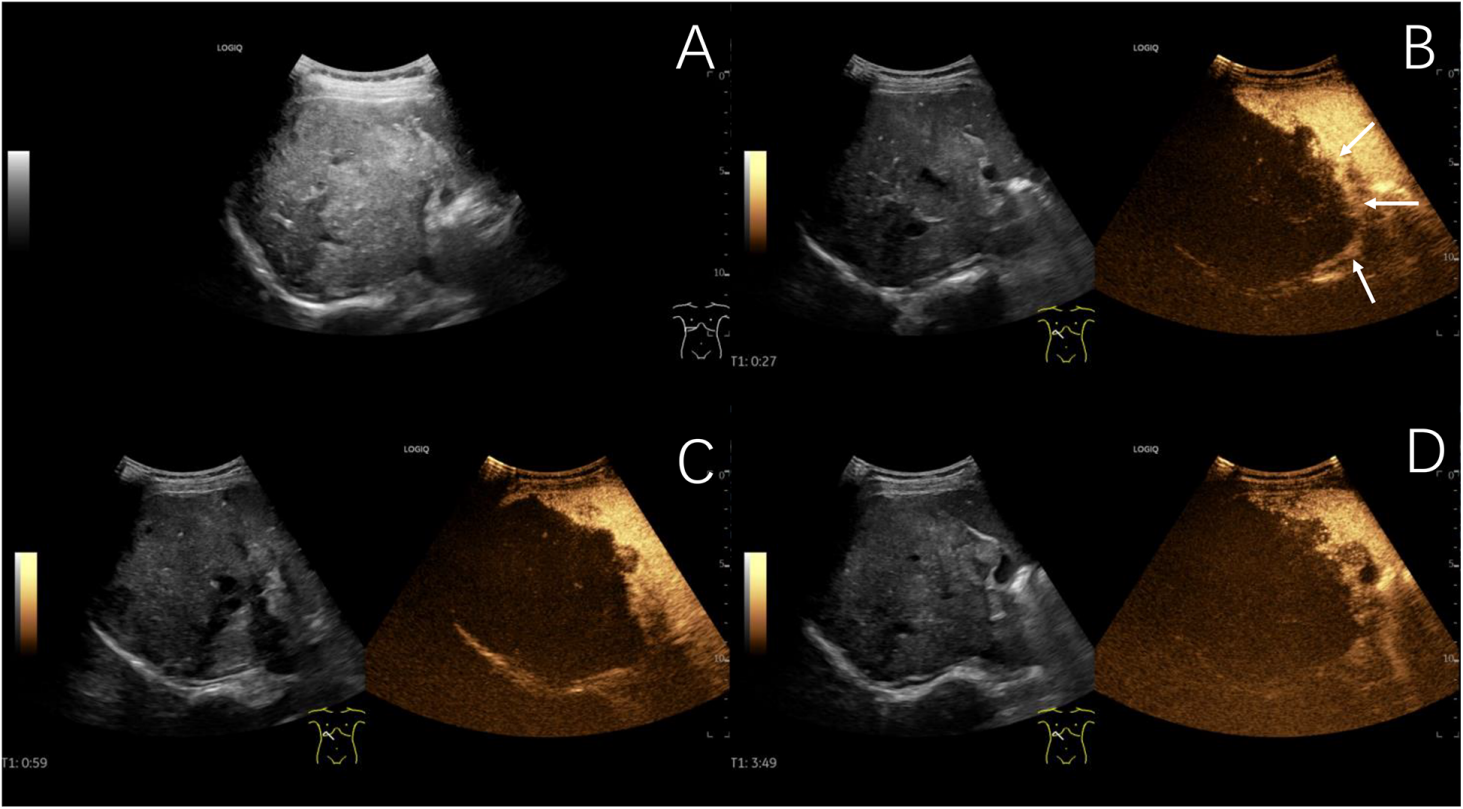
Typical ultrasound findings of hepatic alveolar echinococcosis (case 2). Ultrasound showed a 101 mm hyperechoic mass in the right liver, with unclear boundaries, irregular shape, and patchy strong echoes inside (A) contrast-enhanced ultrasound examination showed slight peripheral enhancement during the arterial phase (B), with no enhancement observed internally in the arterial phase, portal venous phase (C), or late phase (D) (The white arrows indicate the peripheral slightly hyperenhanced sign of the lesion in the arterial phase).
